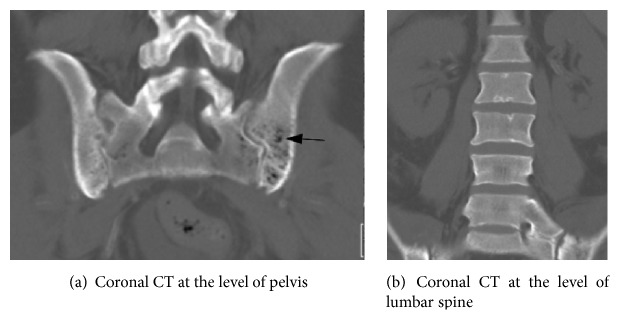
Coronal noncontrast CT three days prior to discharge/approximately 11 weeks after initial presentation shows (a) new foci of gas (arrow) within the sacrum and iliac bones, left greater than right. Findings in keeping with the preceding iliac bone marrow biopsy showing extensive bone necrosis. (b) Spine at this point still remains normal in CT appearance.
